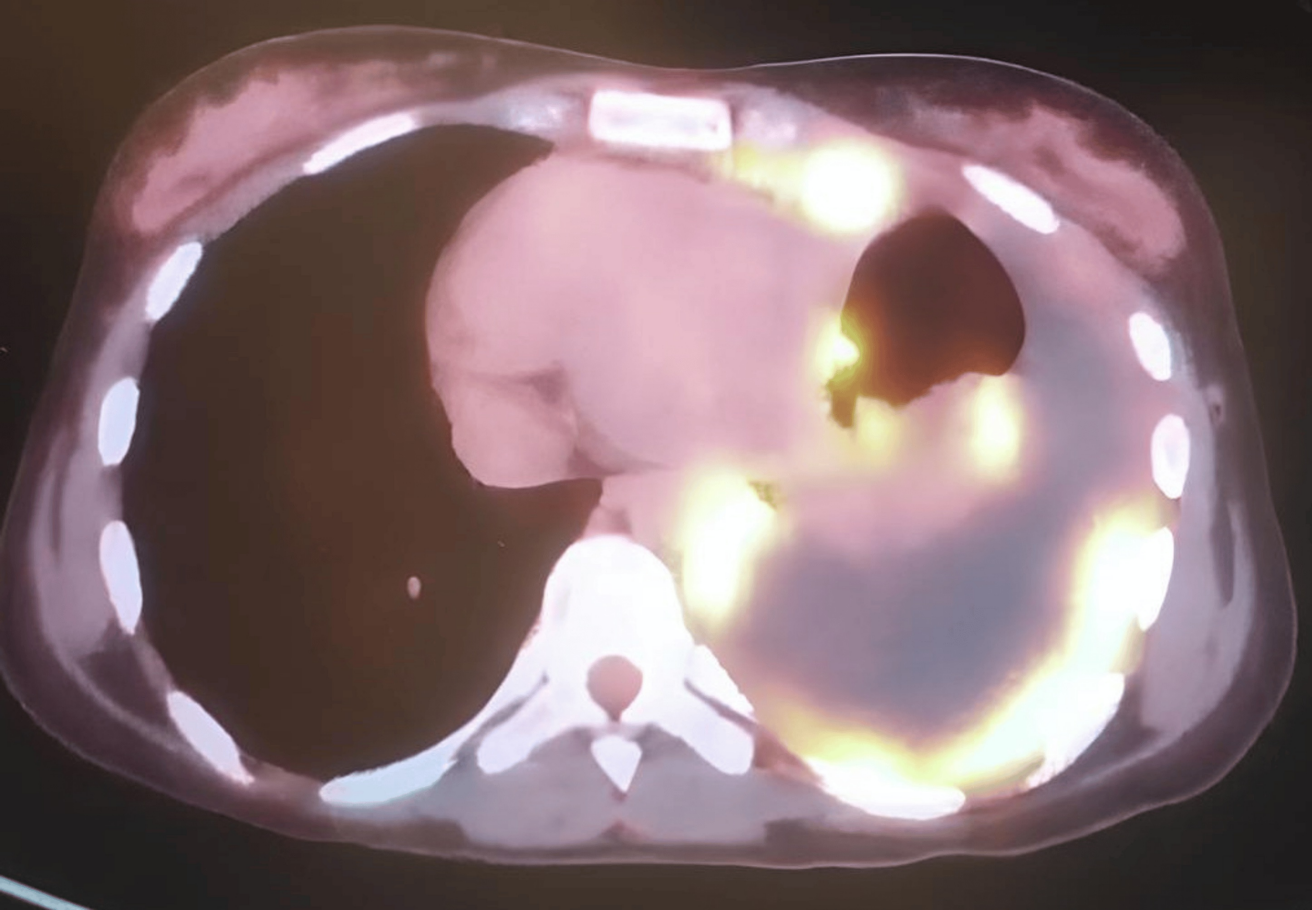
PET-CT scan showing left sided pleural effusion with left pleural enhancement PET-CT - positron emission tomography computed tomography scan
